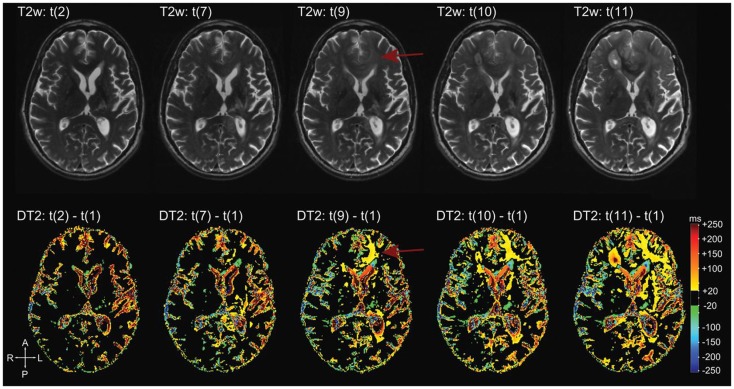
Differential quantitative T2 maps. Reprinted with permission from Ref. (151), License Number 3520001314812. More apparent changes on the differential T2 map (bottom row) in the left frontal lobe (arrows) compared to T2-weighted images (top row). These changes are hardly visible on conventional T2-weighted images (arrows).
